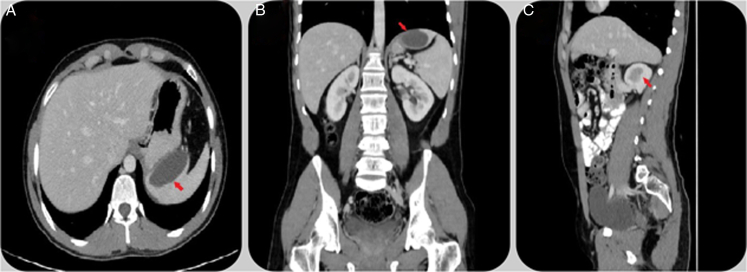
Computed tomography scans of a single splenic hydatid cyst. (A) Axial view showing a unilocular cyst (red arrow). (B) Coronal view with the cyst’s position (red arrow). (C) Sagittal view detailing the cyst’s anteroposterior dimension (red arrow).
